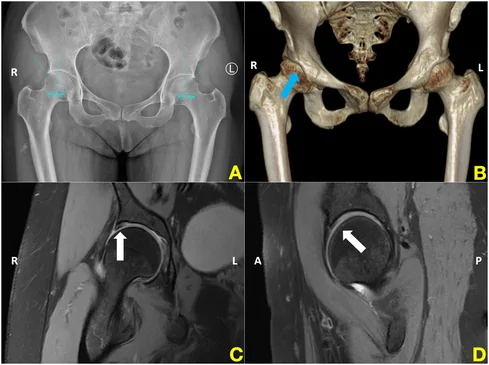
Preoperative imaging examinations. (A) Weight‐bearing pelvic anteroposterior radiograph, showing the lateral center‐edge angle of the right hip is 40°; (B) 3‐dimensional computed tomography reconstruction image, showing excessive coverage of the anterior and lateral acetabulum of the right hip with osteophyte hyperplasia (blue arrow); (C,D) magnetic resonance imaging oblique coronal and oblique sagittal views, showing obvious labral tear in the right hip (white arrow).
